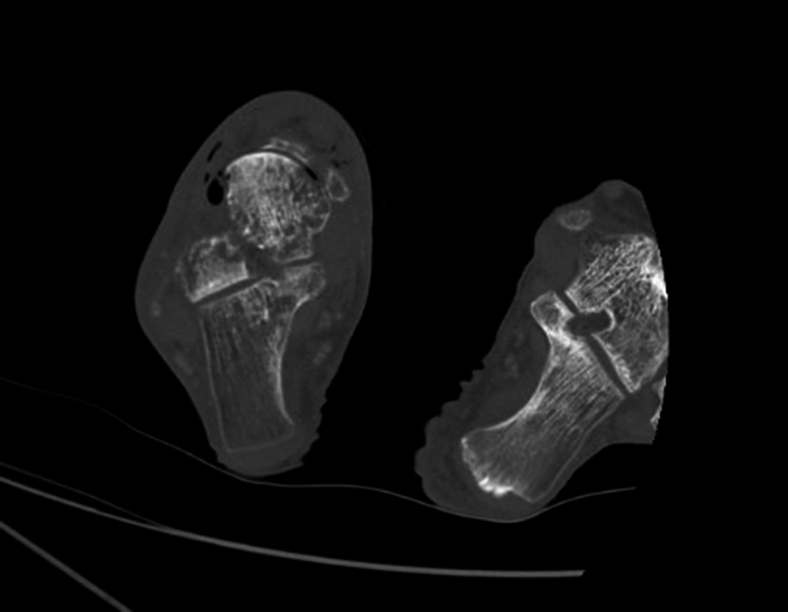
Axial computed tomography right lower extremity with cortical destruction of talar and midfoot bones.
